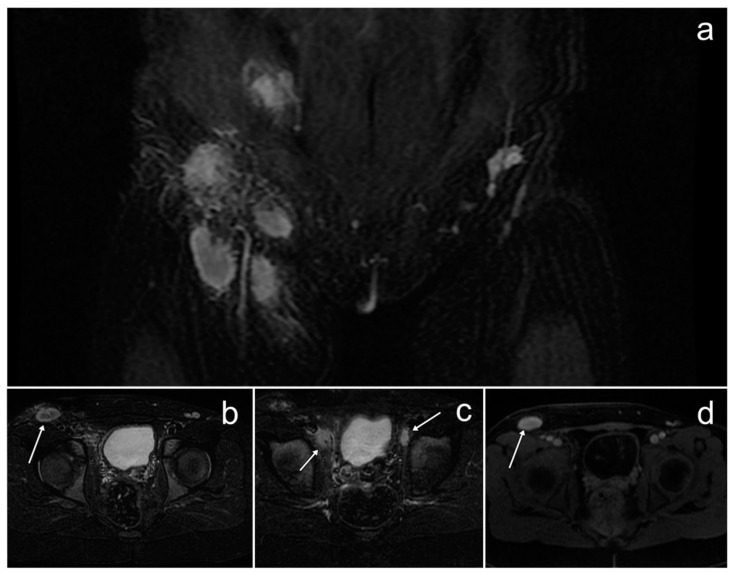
The inguinal and pelvic lymph nodes in Rosai–Dorfman disease on MRI (b–d, arrows). (a–c)—STIR; (d)—post-contrast LAVA/T1 WATER.
